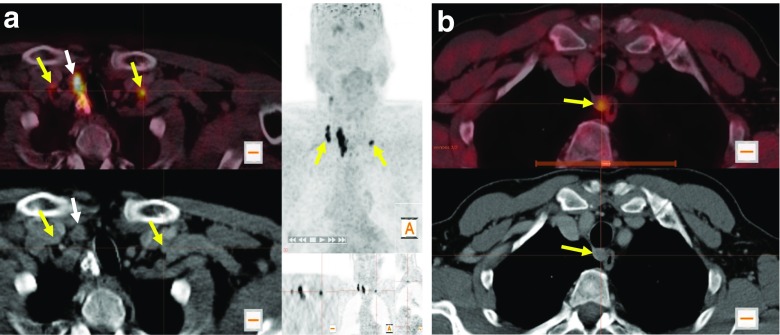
Initial [^18^F]DOPA PET/CT examinations in (a) a 61-year-old male patient with increased DOPA uptake in right and left level IV LNs (*yellow arrows*), while neck ultrasonography showed suspicious lymph nodes only on the right side, and (b) a 72-year-old male patient with increased DOPA uptake in a lymph node at lower level VI that was not been seen with neck ultrasonography
